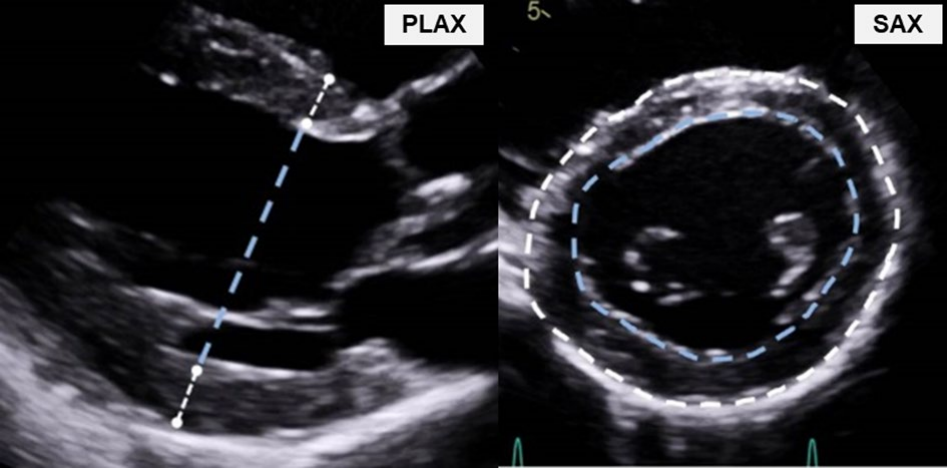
Wall thickness measurements at the chordae level. *PLAX* parasternal long-axis, *SAX* parasternal short-axis.
